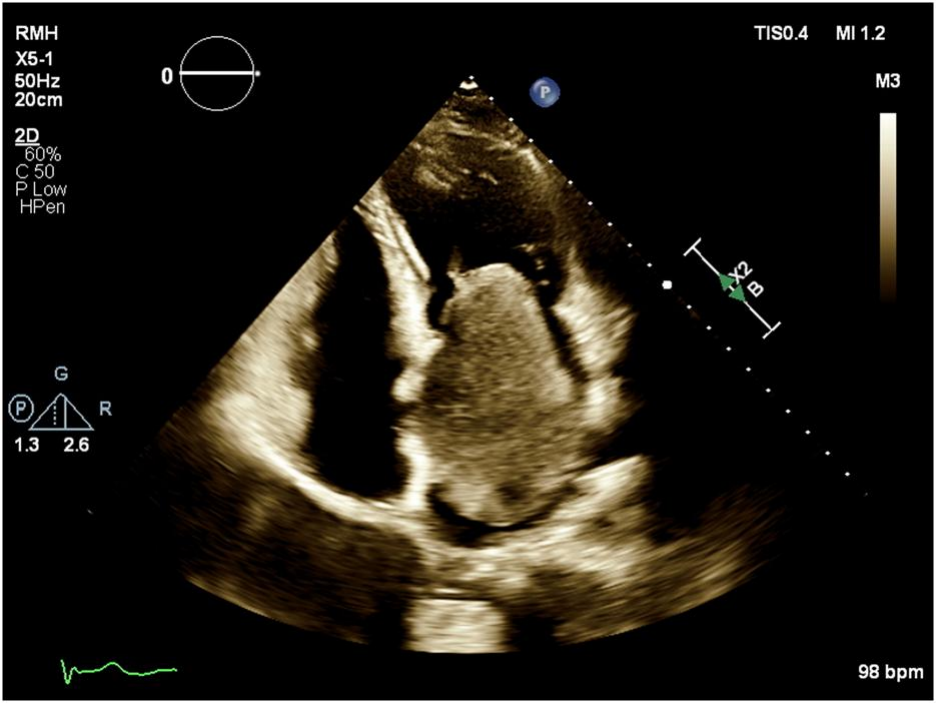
Transthoracic echocardiogram demonstrating an apical 4 chamber view with a massive left atrial mass attached to the fossa ovalis and extending through the mitral valve, causing moderate-to-severe mitral valve obstruction.
